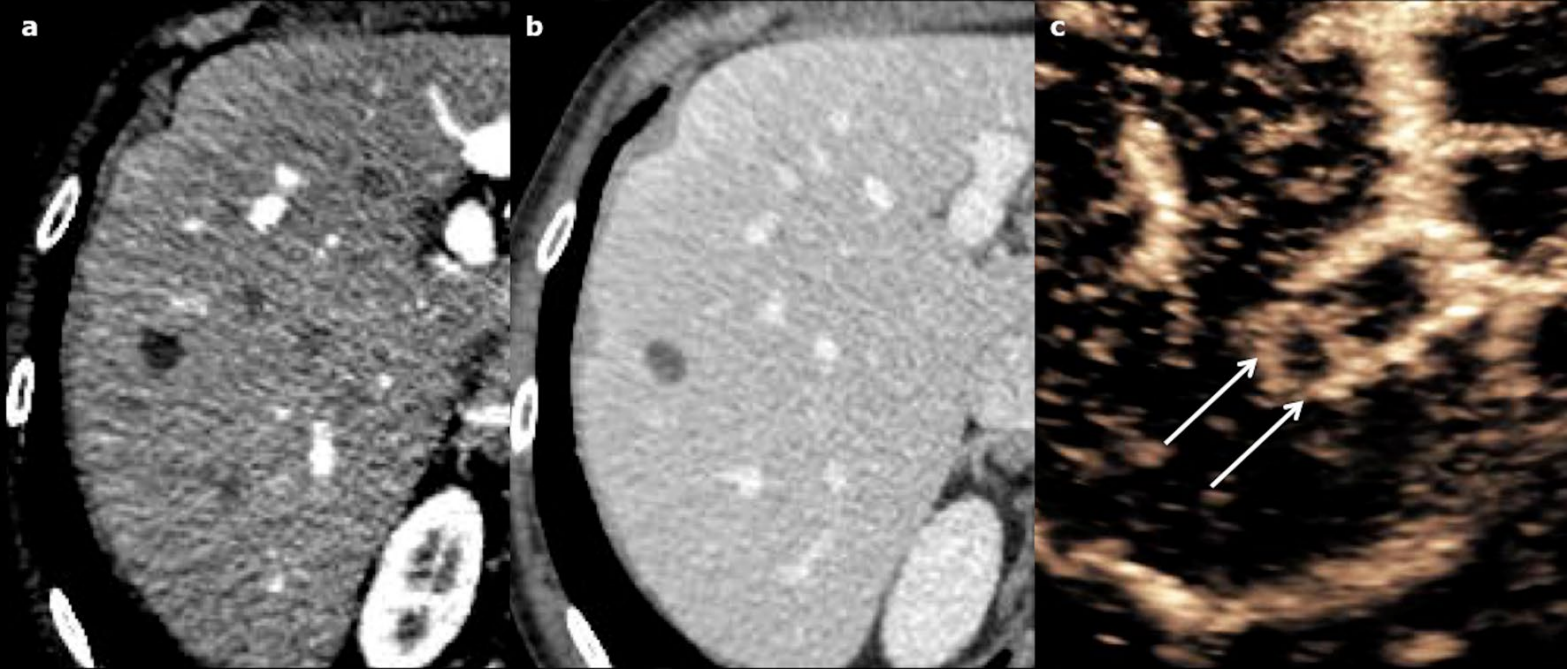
Cystic metastasis from malignant melanoma in a 72-year-old female. a, b Axial computed tomography on arterial and portal venous phases shows unique hypoattenuating (20 Hounsfield Units) nodule of the right liver lobe, too small to be characterised. c Contrast-enhanced ultrasonography displays an enhanced wall as a “rim” (arrows)
